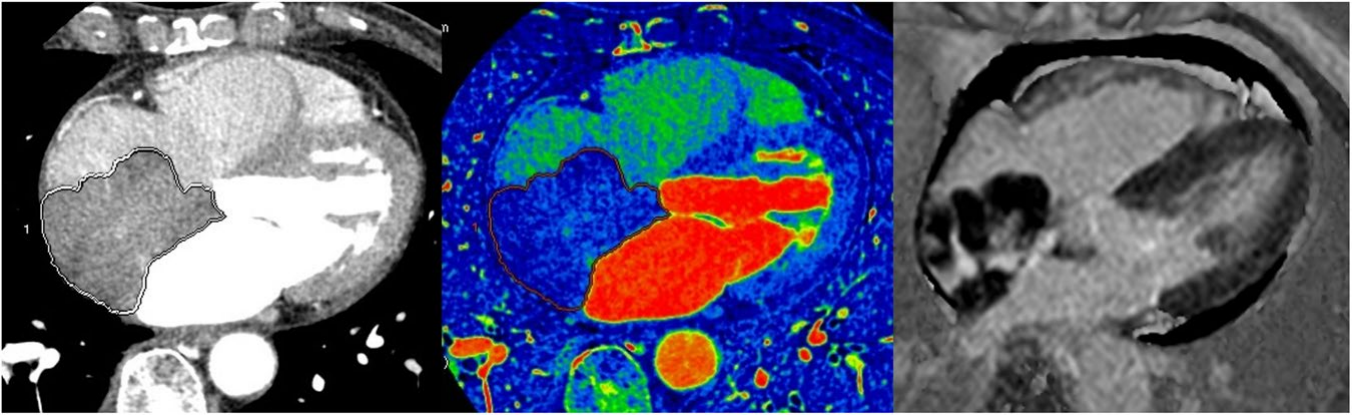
A 72-year-old female patient diagnosed with a lymphoma. (A) On enhanced CT, a 6.8 × 4.8-cm lobulated mass was observed in the right atrium. The volume of the mass was 47.1 cm^3^ and the CT attenuation value of the intra-cardiac mass was 70.0 HU. (B) On the iodine (water) image, the iodine concentration within the VOI was 1.67 mg/ml. (C) MRI was performed three days later. On the LGE image, the mass shows central enhancement, the SI ratio of the mass was 4.16. Minimal pericardial effusion is noted.
